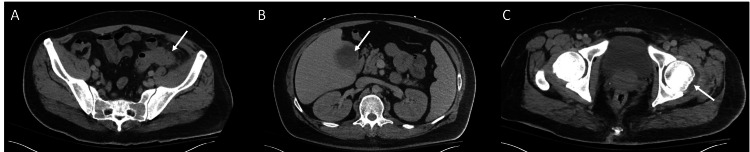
Axial CT of the abdomen and pelvis with contrast showed severe sigmoid diverticulitis with hepatic fluid collection and small left hip joint effusion. (A) Sigmoid diverticulitis with associated fat stranding; (B) Hepatic abscess; (C) Small left hip joint effusion; Arrows point to respective processes (A-C).
